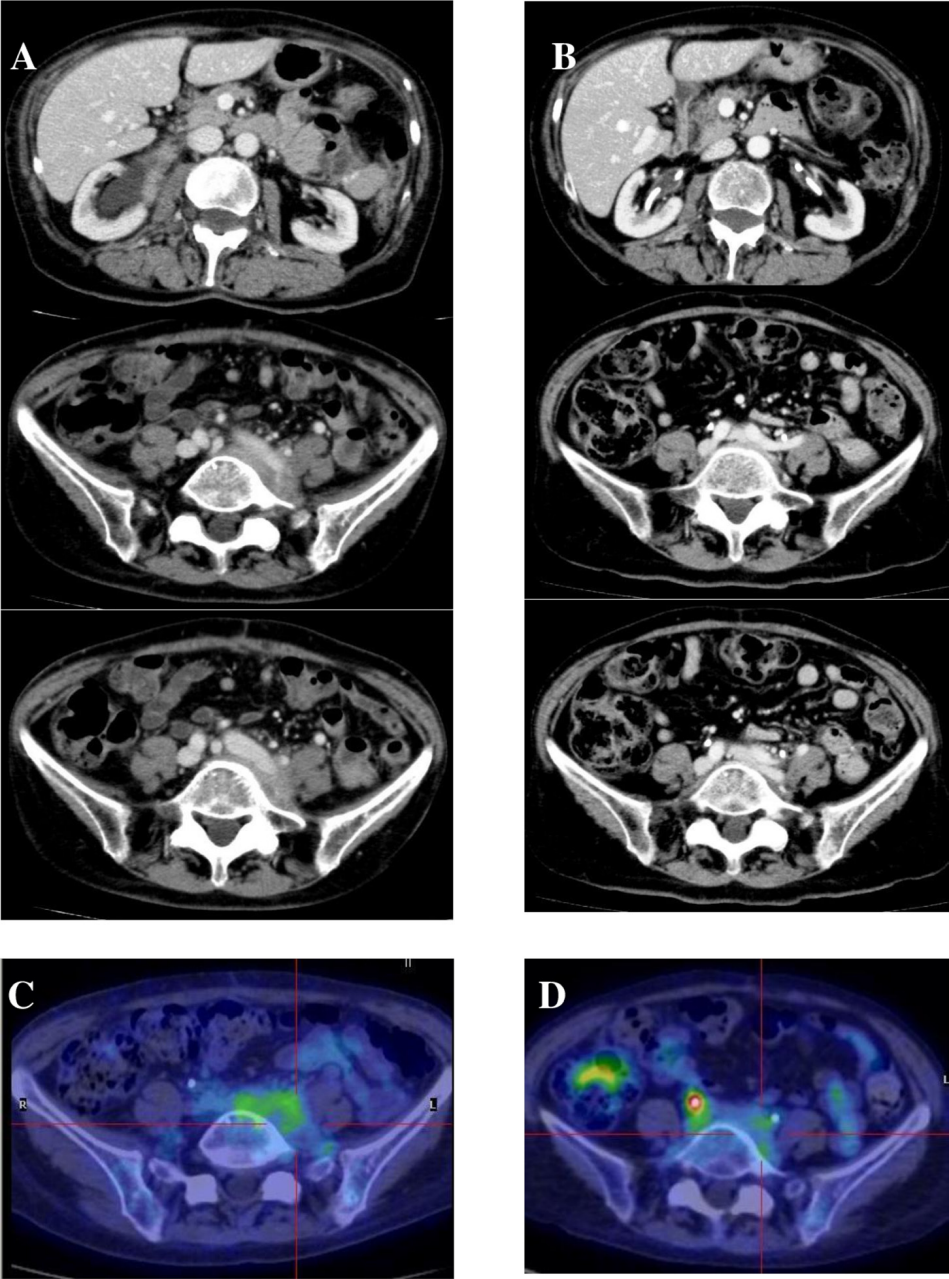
Radiologic features of patient #5 CT scan and PET/CT imagings before starting therapy (A, C) and at 6 months (B, D): (A) retroperitoneal and perivascular fibroinflammatory tissue, with (C) high metabolic activity; (B) complete disappearance of abnormal tissue, with (D) persistence of residual minimal pelvic metabolic activity.
